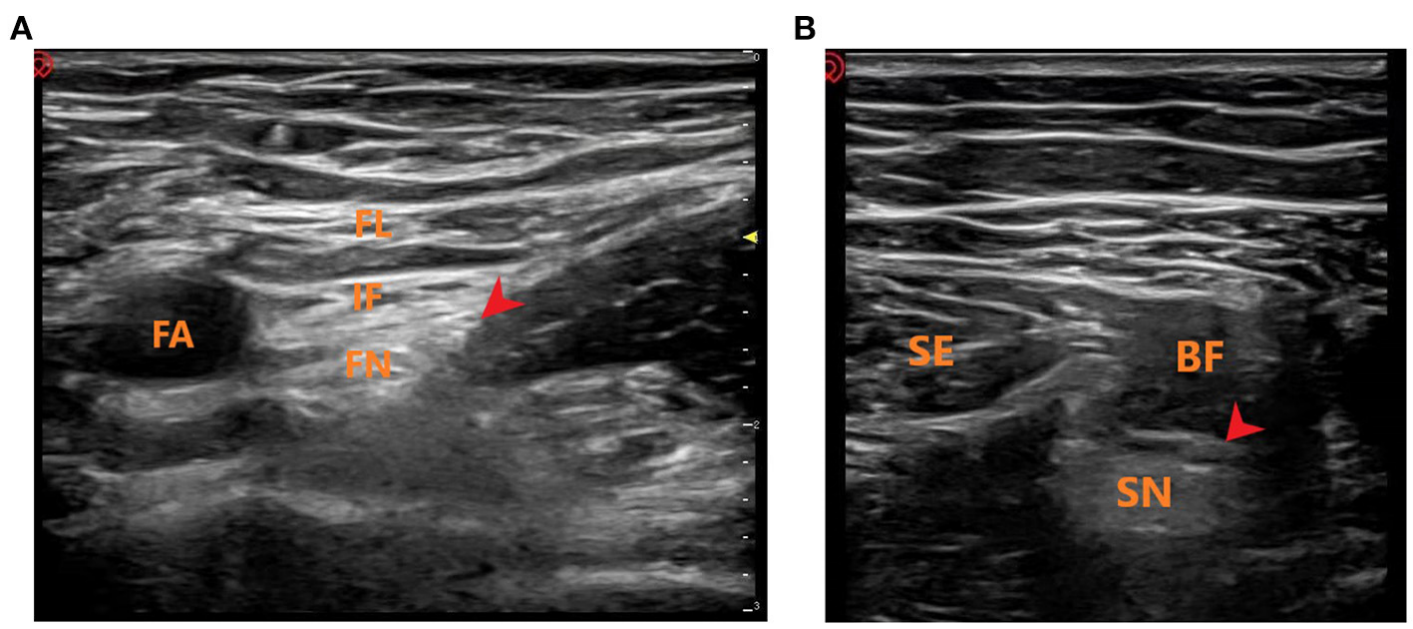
Ultrasound-guided femoral and sciatic nerve block. (A) Ultrasound-guided femoral nerve block. FL, fascia lataI; IF, iliac fascia; FA, femoral artery; FN, femoral nerve; Red Arrow: Local anesthetic injection site. (B) Ultrasound-guided sciatic nerve block. SE, semitendinosus; BF, biceps femoris; SN, sciatic nerve; Red Arrow: Local anesthetic injection site.
